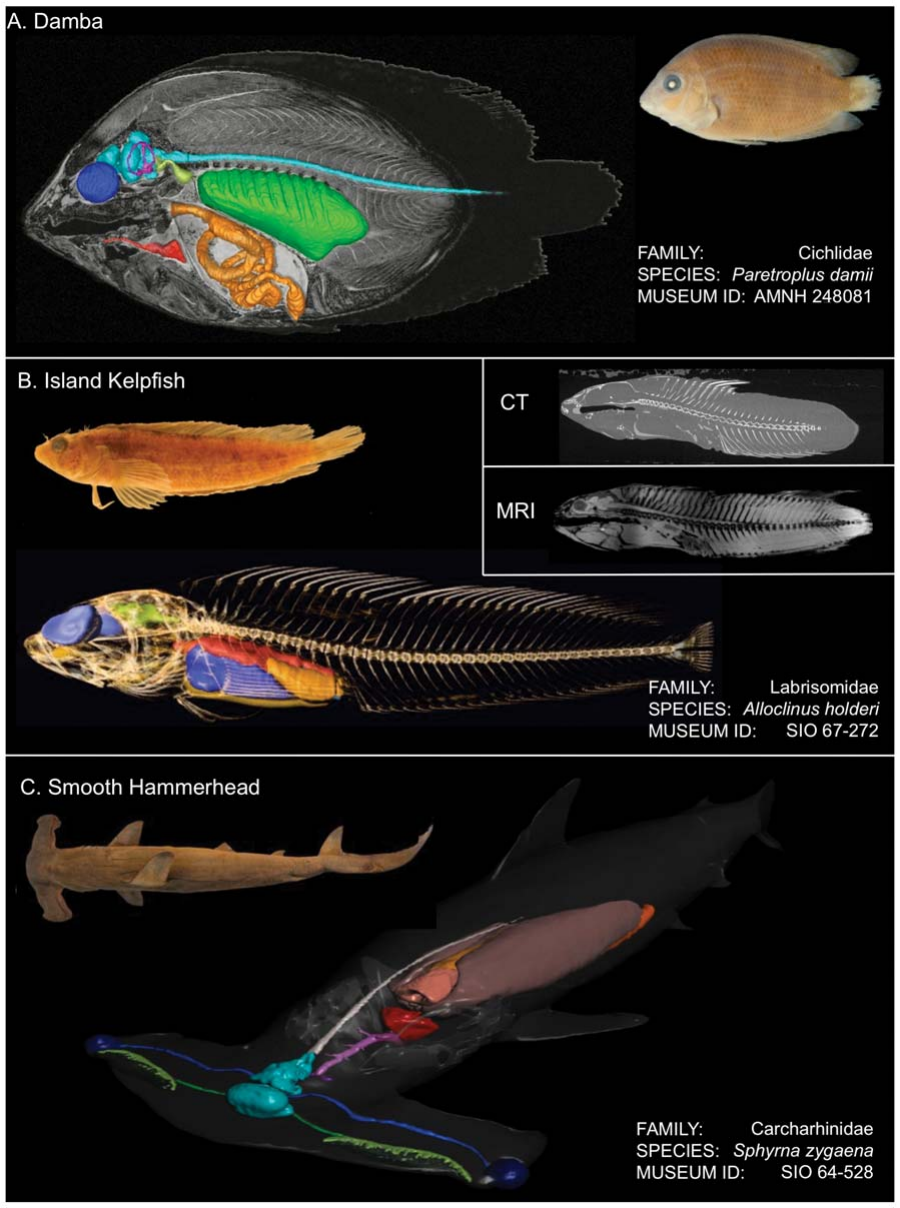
Examples of 3D segmentation and rendering of DFL data. (A) This damba, *Paretroplus damii* (AMNH 248081, SL 76 mm) was imaged at 7*T* with the following 3D FLASH pulse sequence parameters: FA = 15°, TR = 24.07 ms, TE = 11.92 ms, slice thickness = 100 µm, slice matrix = 300×1024×500, resolution = 100 µm^3^, averages = 2. MRI slice data is overlaid with a selection of segmented soft tissue structures color-coded as follows: light blue = brain and spinal cord; dark blue = eyes; magenta = vestibular labyrinth; green = gas bladder; light brown = alimentary tract; red = heart and dorsal aorta. (B) Both MRI and CT data were acquired for this island kelpfish, *Alloclinus holderi* (SIO 67-272, SL 94 mm). A selection of soft tissue structures were segmented from MRI data and are color-coded as follows: blue = eyes; green = brain; red = alimentary tract; light brown = ovaries; purple = liver. Segmented soft tissues were co-registered with a volumetric rendering of CT imaged hard tissue structures acquired by The University of Texas High-Resolution X-ray Computed Tomography Facility (UTCT) (slice thickness 42 µm). (C) This smooth hammerhead, *Sphyrna zygaena* (SIO 64-528; SL 104 mm) was imaged at 3*T* with the following 3D FSPGR pulse sequence parameters: FA = 35°, TR = 9.86 ms, TE = 4.11 ms, slice thickness = 900 µm, slice matrix = 512×512×236, resolution = 683 µm^3^, averages = 2. It was scanned in 4 sections and pieced together in Amira for segmentation. A selection of segmented soft tissue structures are color-coded as follows: brown = alimentary tract; dark blue = eyes; green = olfactory system; light blue = brain; magenta = dorsal aorta; puce = liver; red = heart; white = spinal cord.
